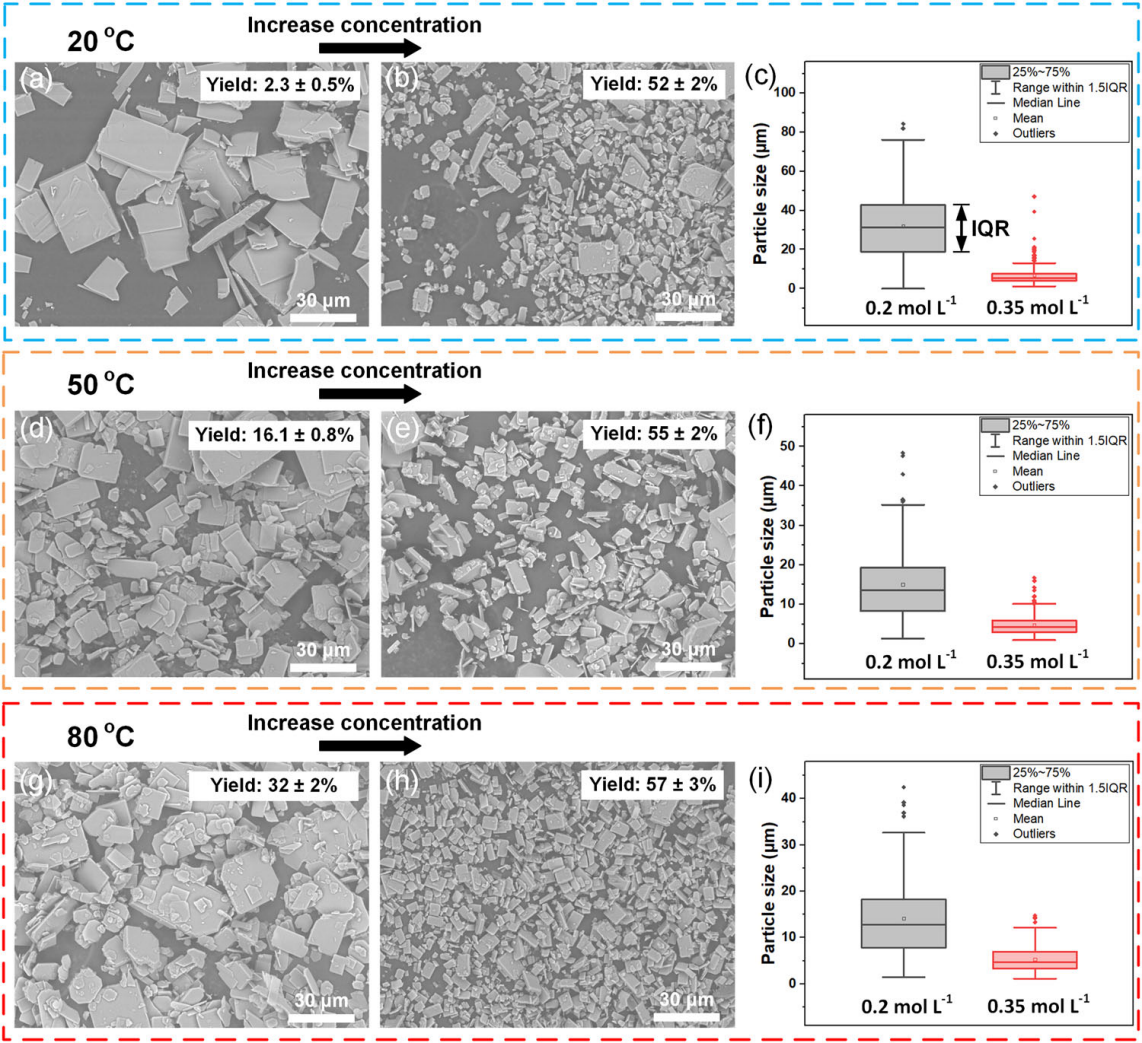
SEM images of Ca-NDS (water) made in single-phase ultrasound-assisted flow. SEM images depict Ca-NDS (water) prepared across (a)–(c) 20 °C, (d)–(f) 50 °C, and (g)–(i) 80 °C. Each row contains images for concentrations of precursor solutions with the same metal-to-ligand ratio at (a), (d), (g) 0.2 M and (b), (e), (h) 0.35 M as well as (c), (f), (i) the corresponding box plots showing the distribution of particle sizes. The horizontal lines in the box plots are the minimum, first quartile, median, third quartile, and maximum. The mean is shown as a square. Outliers (greater than 1.5 times the interquartile range) are shown as additional points. The residence time of these reactions was 1.25 min.
